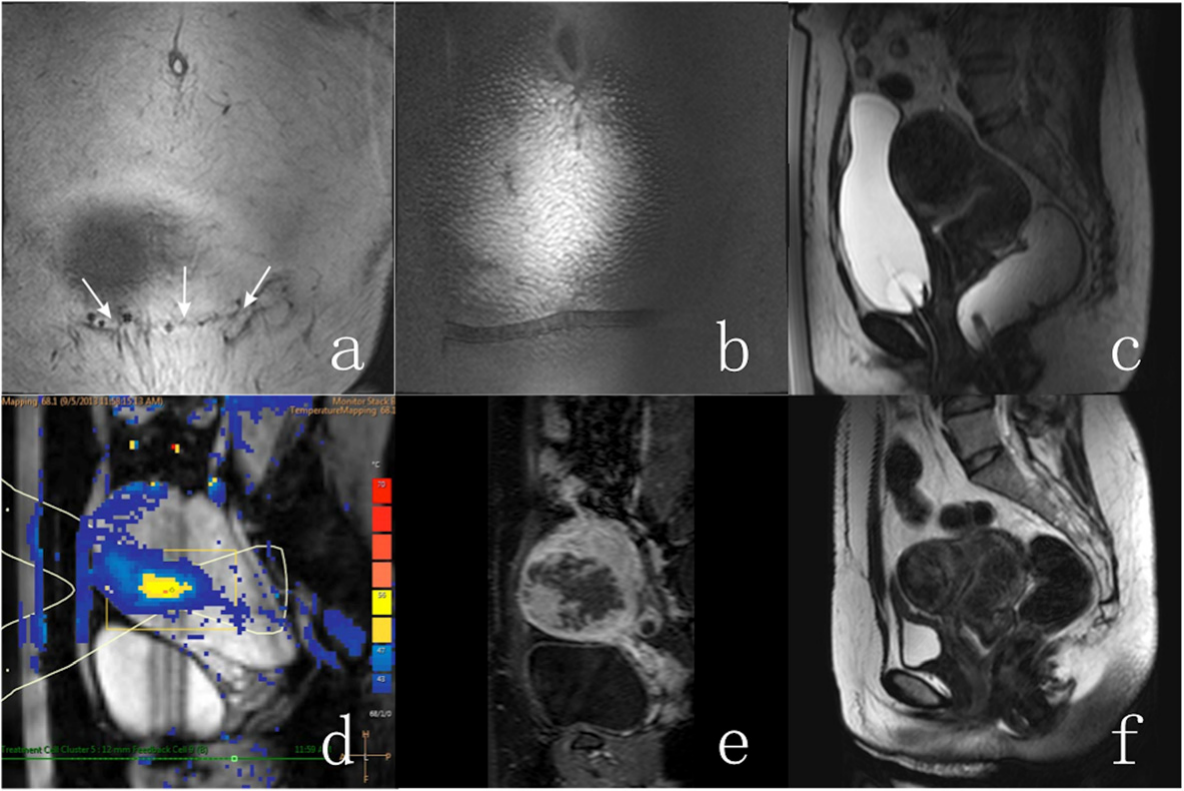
A 41-year-old woman who had a transverse incision scar presented with symptoms of menorrhagia, pelvic pressure, and urinary frequency was treated with MRI-guided high-intensity focused ultrasound ablation using scar patch. a Scar scan showing the orientation of the scar within the abdominal fat layer (scar location identified with white arrows). b Scar scan showing the air-containing scar patch at the patient’s skin. c T2w planning image acquired prior to the therapy. d PRF Thermometry scan acquired during one of the sonications. e T1w gadolinium-enhanced image acquired immediately after the therapy showing the induced necrosis. f T2w planning image at 6-month follow-up
